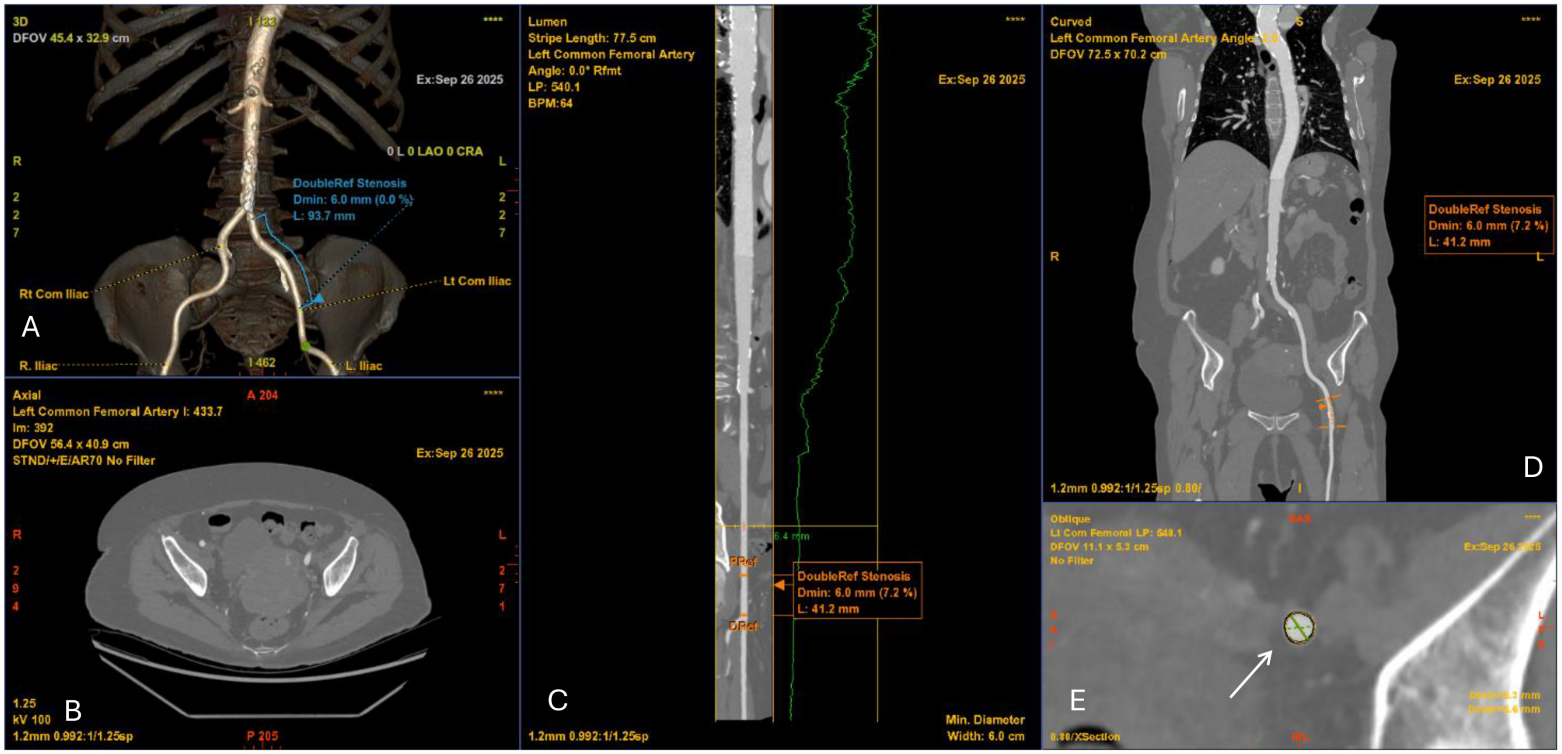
Computed tomography angiography (CTA) of the iliofemoral vessels
